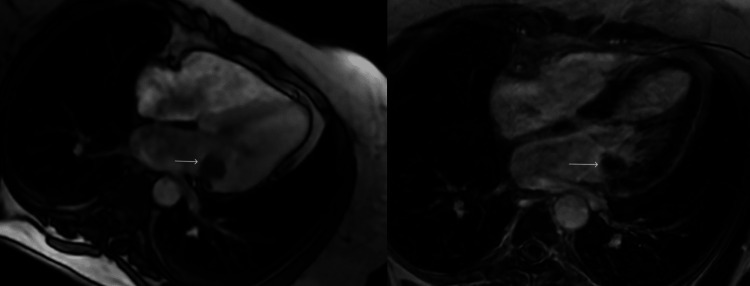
Axial T2 bright blood image and post-contrast T1-weighted image demonstrating a T2 hypointense non-enhancing focus of calcification corresponding to the echogenic focus seen on echocardiography (white arrow).
